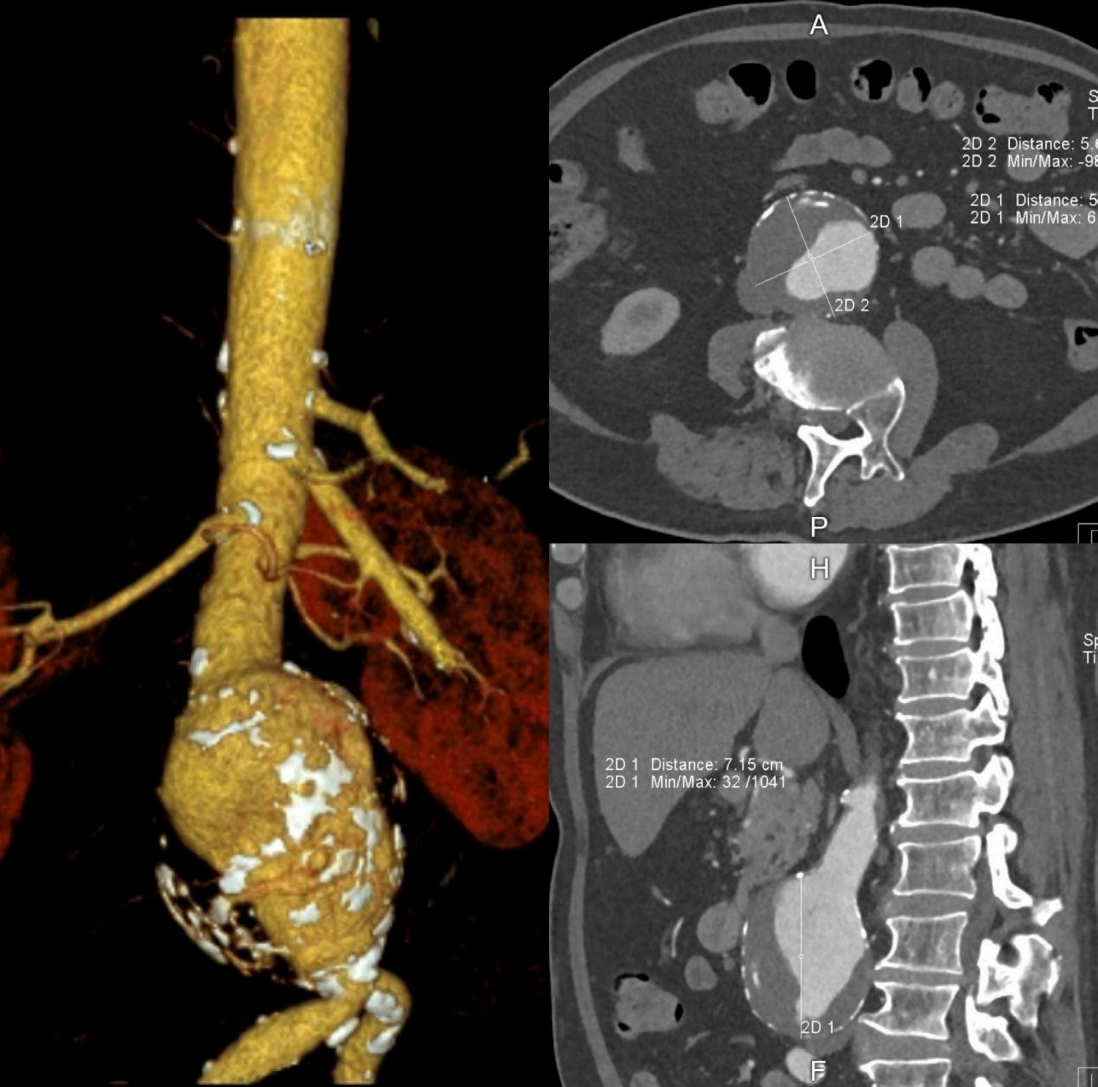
Computed tomography abdominal aortogram measured a 7.15 cm × 5.67 cm × 5.46 cm diameter abdominal aortic aneurysm.
